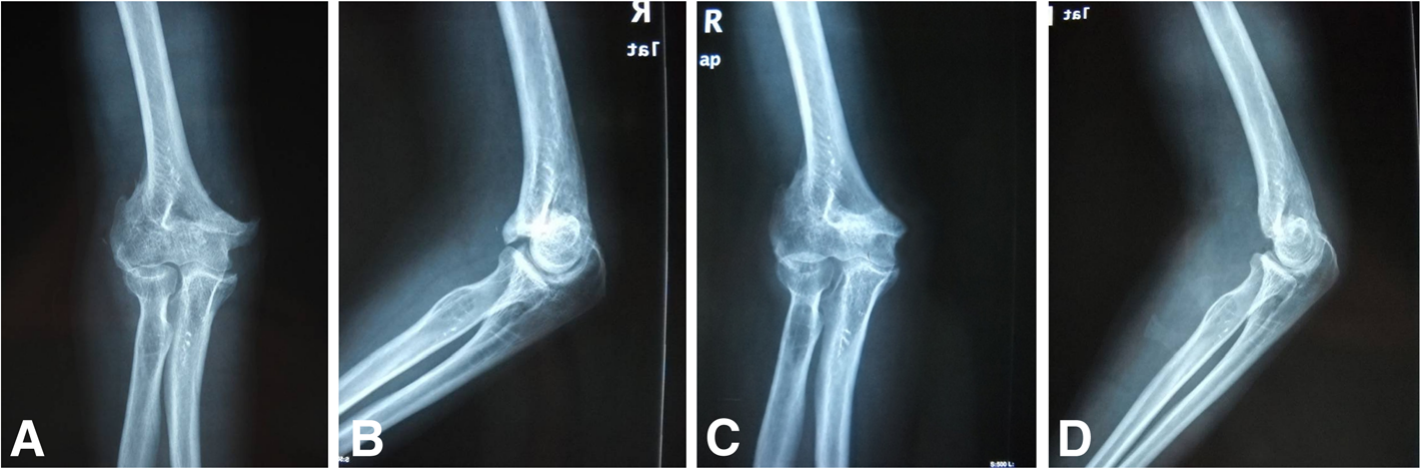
a-d Preoperative and postoperative radiographs of a 41-year-old male with 8 months old fracture. (a) and (b) are preoperative radiographs of anteroposterior (AP) and lateral view respectively; and (c) and (d) are postoperative radiographs. The ROM was improved significantly from 30° to 60° preoperatively to 10° to 110° postoperatively
